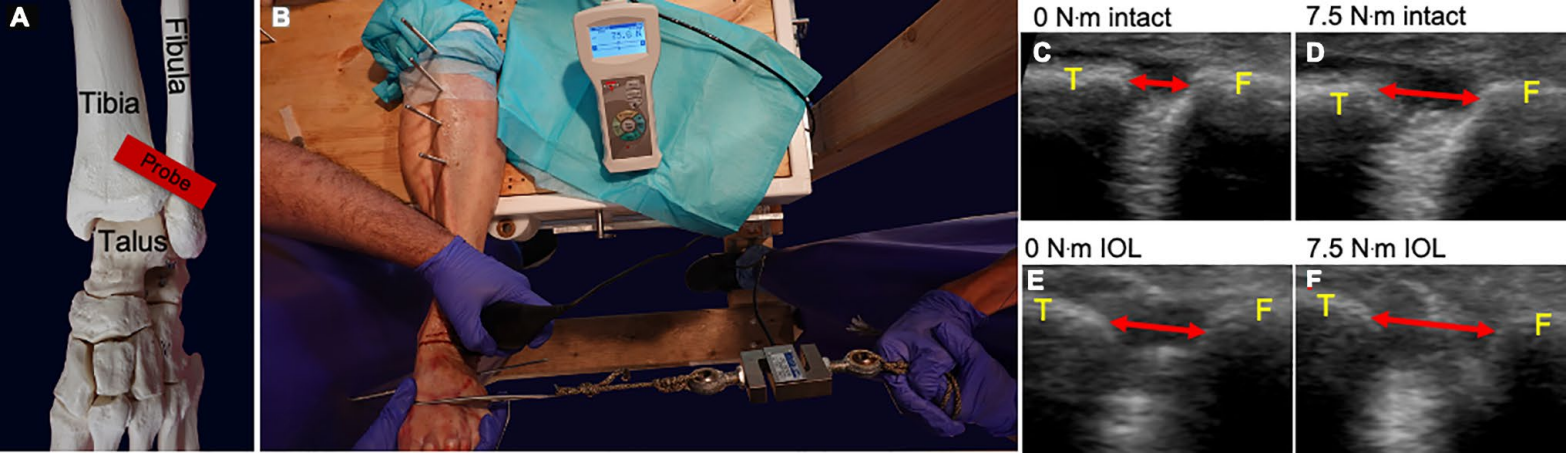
P-US experimental setup. A Bone model of probe position. B Ultrasound experimental set up. C–F Ultrasound image of the TFCS at 0 and 7.5 Nm, at the intact stage (C, D) and after AITFL + IOL transection (E, F). Red arrows resemble the TFCS measurements. *T* tibia, *F* fibula
